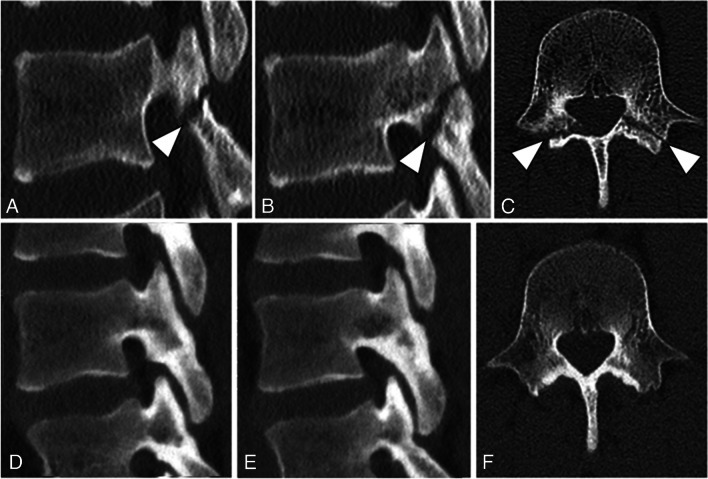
A Sagittal base-line CT image on the left side of a 15-year-old adolescent with a spondylolysis of L4 (arrowhead). B Sagittal CT image on the right side with a spondylolysis at the same level (arrowhead). C Axial CT image of the same patient with the bilateral spondylolysis (arrowheads). D Sagittal follow-up CT image of the same patient (now 23 years old) with fused lysis gap on the left side, and E sagittal follow-up CT image on the right side with fused lysis gap. F Axial follow-up CT image of L4 in the same patient with bilateral fused spondylolysis
